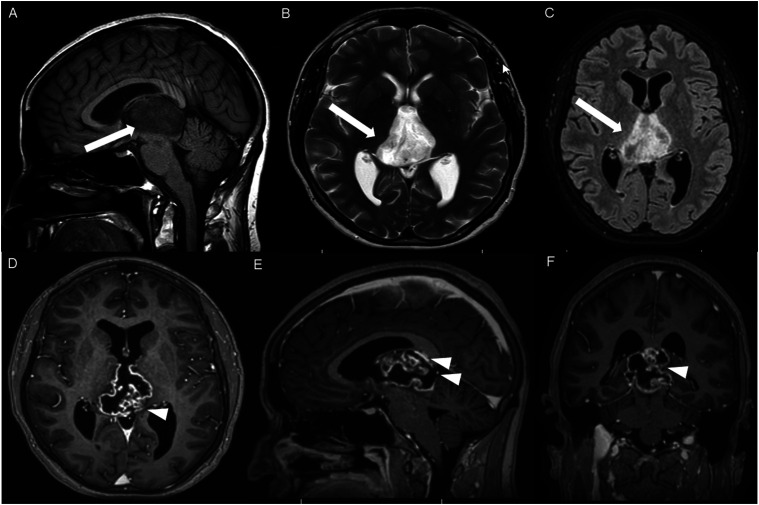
Sagittal T1 weighted image (A), axial T2 weighted image (B), axial FLAIR (C), axial post-contrast T1 weighted image (D), sagittal post-contrast T1 weighted image (E) and coronal post-contrast T1 weighted image (F) show a T1 hypointense, T2/FLAIR hyperintense mass involving the third ventricle and medial thalami (A-C, arrows) with peripheral irregular enhancement (D-F, arrowhead). There is obstruction at the level of the foramen of Monro, with hydrocephalus.
